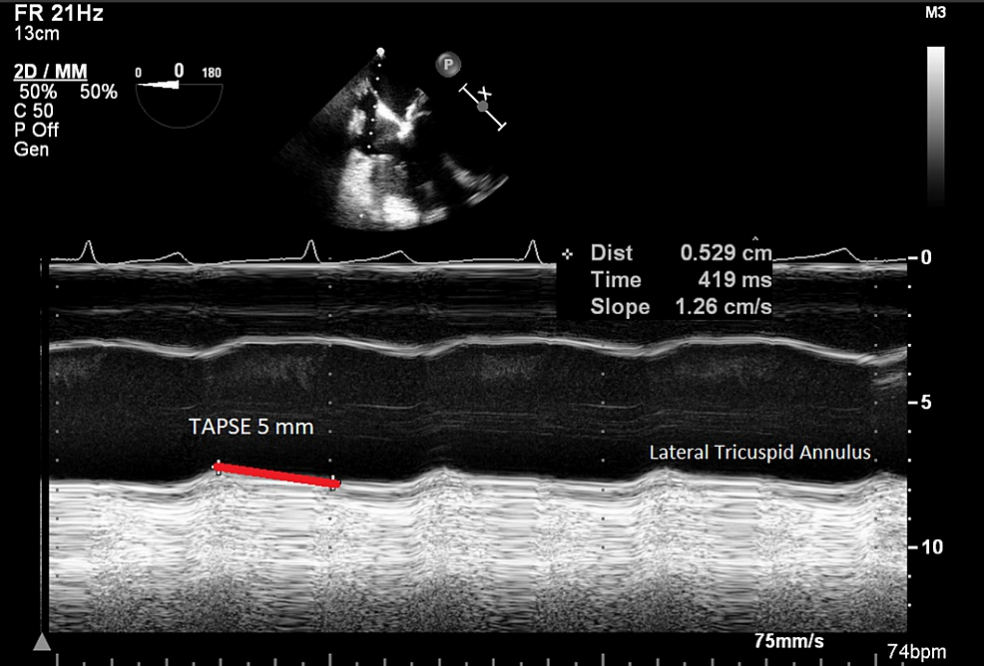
Transoesophageal echo using M-mode to measure Tricuspid Annular Plane Systolic Excursion (TAPSE) (red line) showing severe right ventricular dysfunction.
